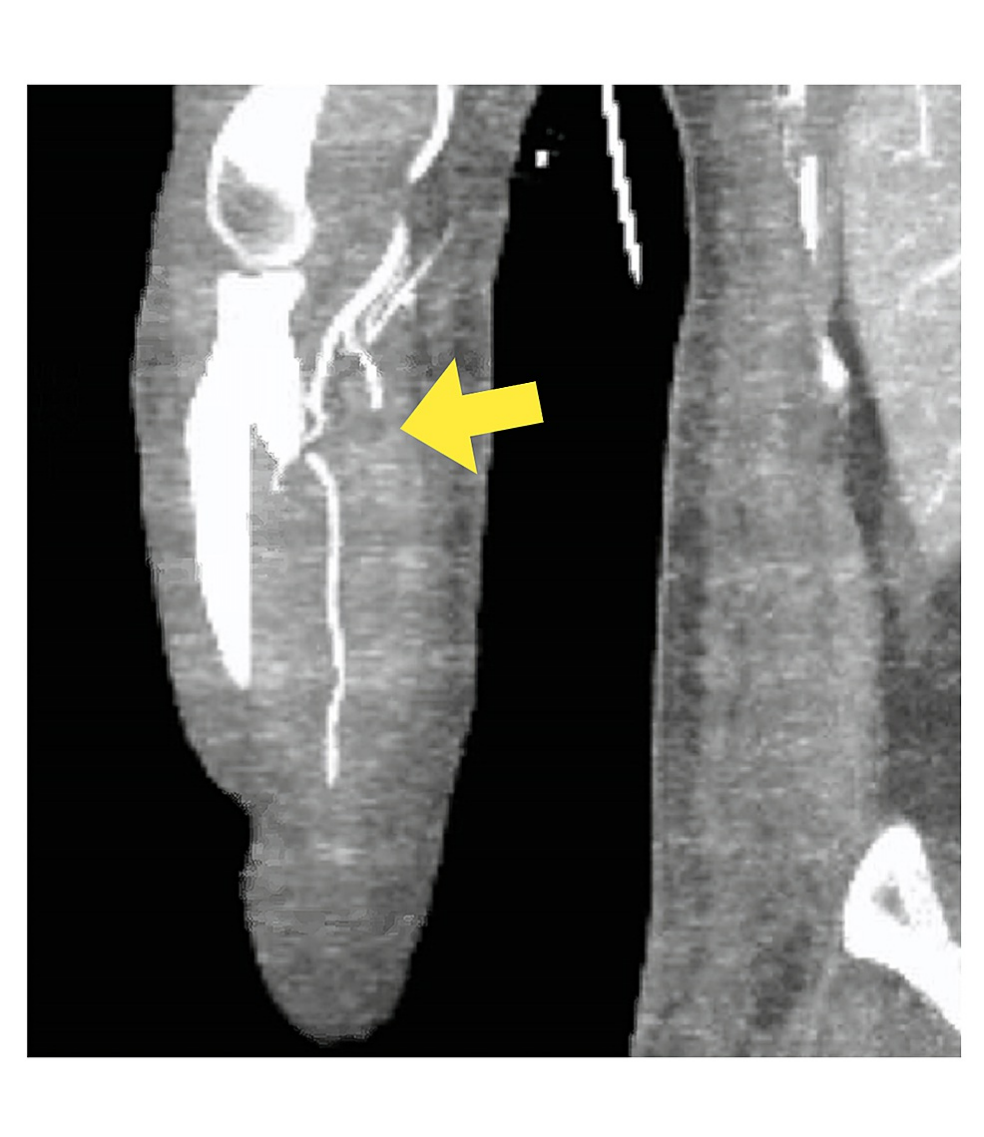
Computed tomography of the right upper extremity showing an occluded right radial artery
